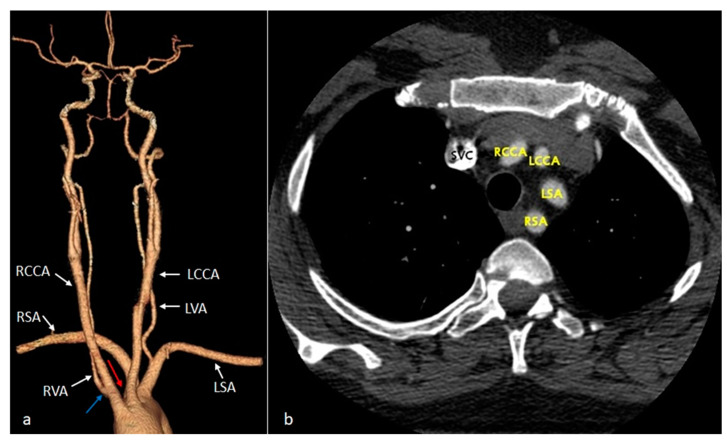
VR (a) and axial (b) CT images showing the aortic arch with the absence of the brachiocephalic trunk and the direct emergence from this level of the four arterial branches. Note the origin of the RSA from the posterior wall of the aortic arch (red arrow), and the RVA from the RCCA (blue arrow). RCCA—right common carotid artery. LCCA—left common carotid artery. LSA—left subclavian artery. RSA—right subclavian artery. SVC—superior vena cava. LVA—left vertebral artery. RVA—right vertebral artery.
